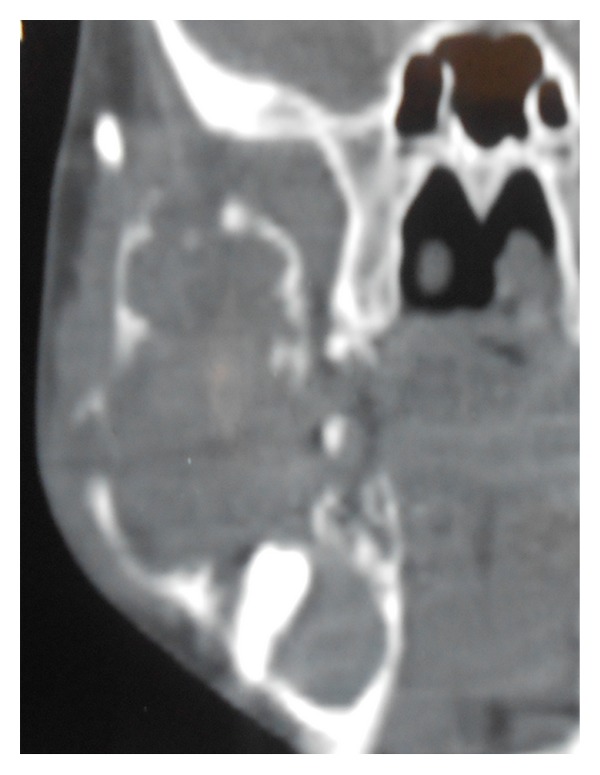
CT scan of the patient showing bicortical expansion with perforation.
